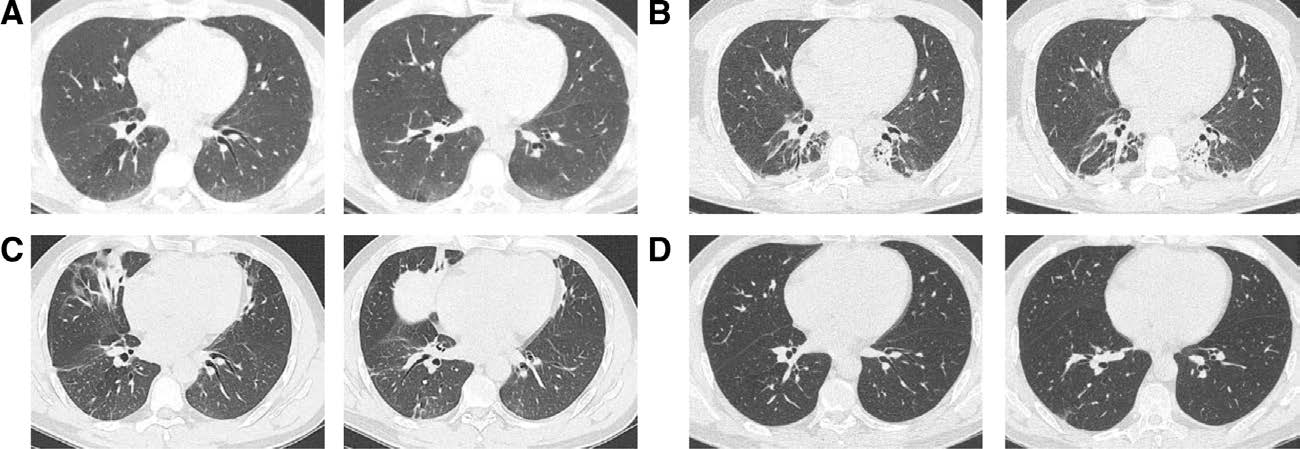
(A)The chest computed tomography scans at admission showed ground-glass opacities in the lower lobes of both lungs. (B) The patient’s condition worsened on the second day of hospitalization, with patchy high-density shadows seen in the lower lobes of both lungs. (C) The patient’s lower lung lobe lesions have been mostly absorbed, and a new lesion has developed in the right middle lung lobe. (D) One month after discharge, follow-up showed that the lesion was basically absorbed, with only a small amount of string-like high-density shadow.
